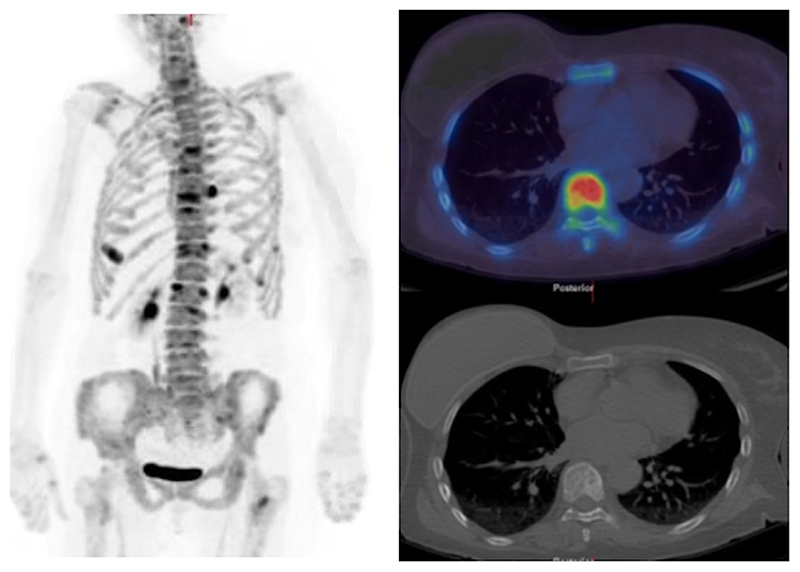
A woman with metastatic breast cancer. The ^18^F-FDG PET/CT scan (a) (maximum intensity projection image - left, axial images through a thoracic vertebra - right) shows only low-grade accumulation in sclerotic metastatic disease. The corresponding ^18^F-fluoride PET/CT scan (b) shows much higher activity reflecting the osteoblastic nature of the metastatic disease in this patient.
